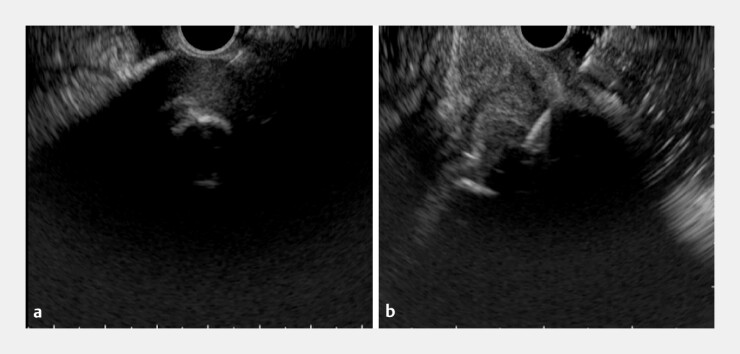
Endoscopic ultrasound (EUS) and EUS-guided fine-needle aspiration (EUS-FNA) findings for the pelvic mass.
